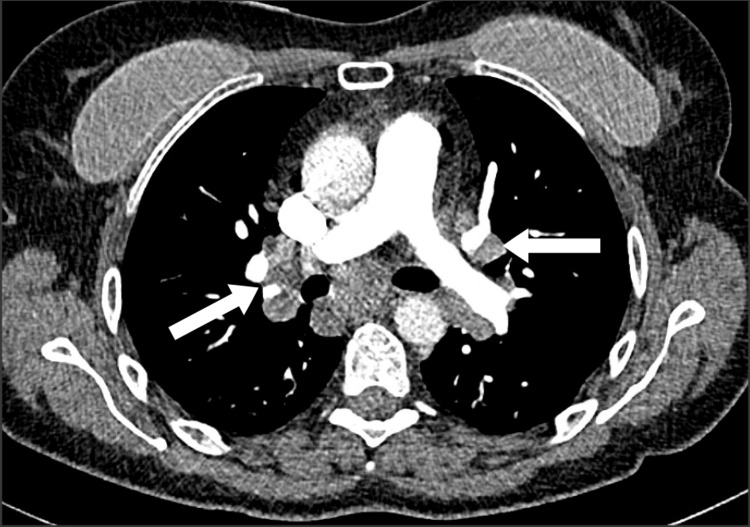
CT scan of mediastinal and bilateral hilar lymphadenopathy
